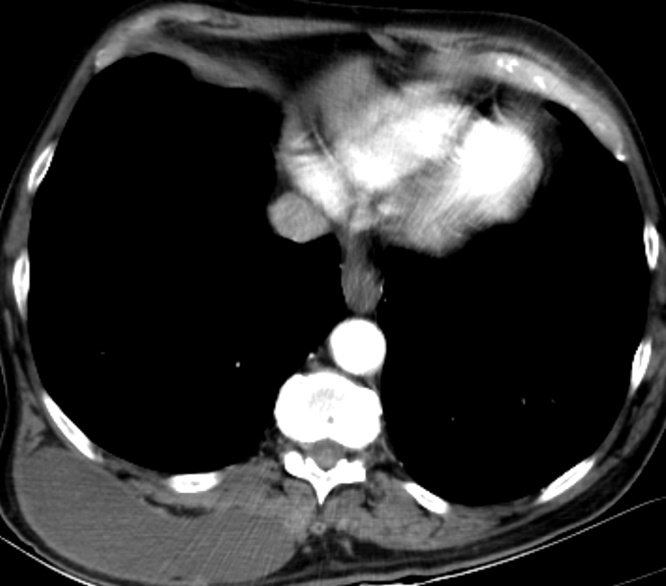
CT Scan of the Chest showing a 10 cm × 10 cm cystic lesion in the right thoracic region.
